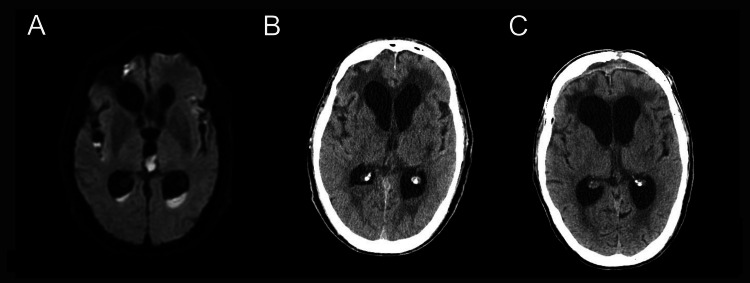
Imaging findings in Case 1 A: Brain MRI-DWI obtained prior to admission shows high signal intensity in the ventricles and subarachnoid space, consistent with intraventricular and subarachnoid abscesses. B: Head CT on admission. C: Head CT at discharge. CT, computed tomography; MRI, magnetic resonance imaging; DWI, diffusion-weighted imaging
